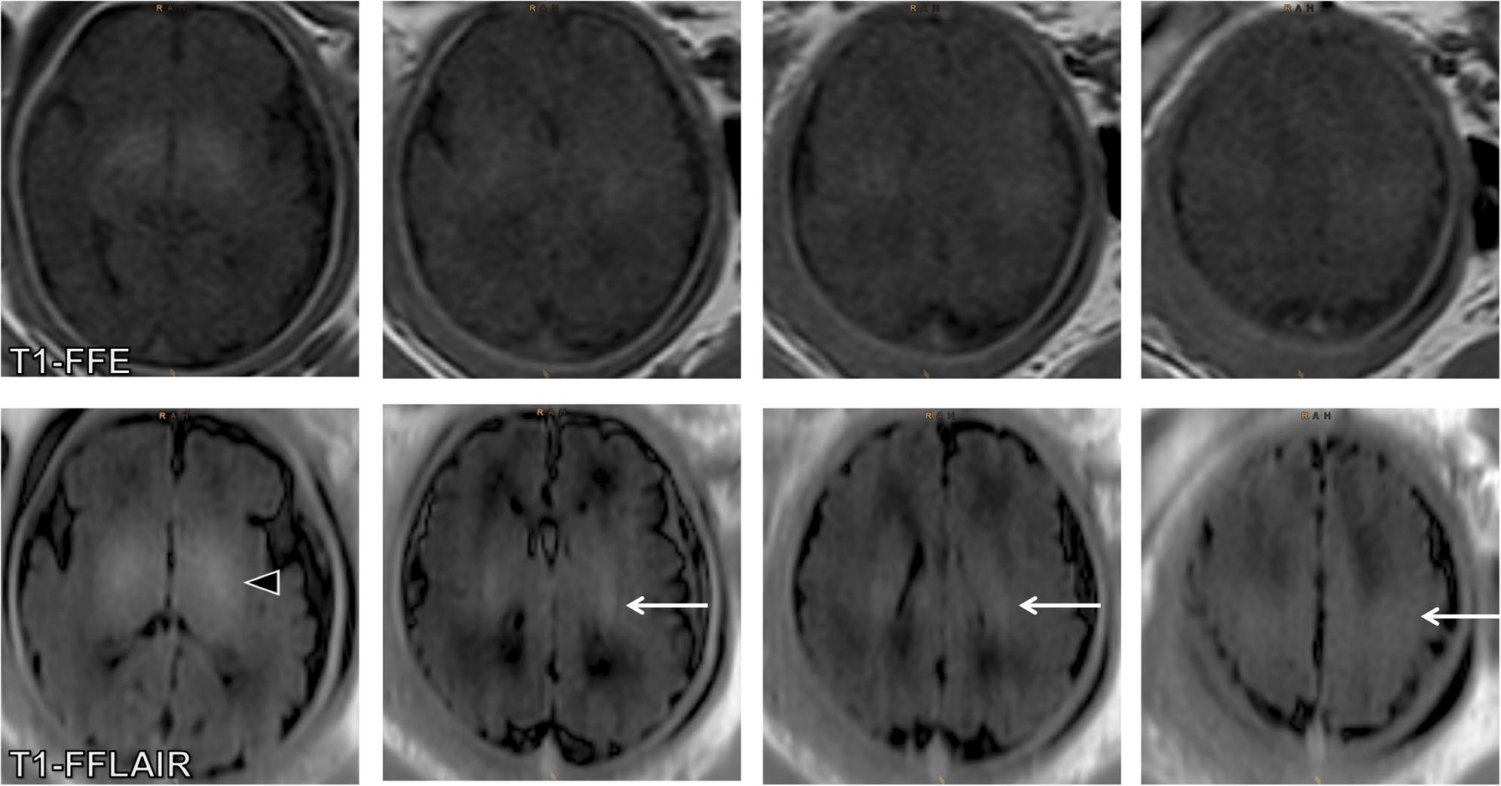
Axial images from T1-fast field echo sequence (T1-FFE) (*upper row*) and T1-weighted fast fluid-attenuated inversion-recovery (T1-FFLAIR) (*lower row*) sequences at gestational age (GA) 37, showing the clearly hyperintense signal in the globus pallidus (*arrowhead*), as well as the corona radiata and the central region (*arrows*) on the T1-FFLAIR sequence. On the T1-FFE sequence, the signal of these structures is only slightly hyperintense and the corona radiata is only partially identified
